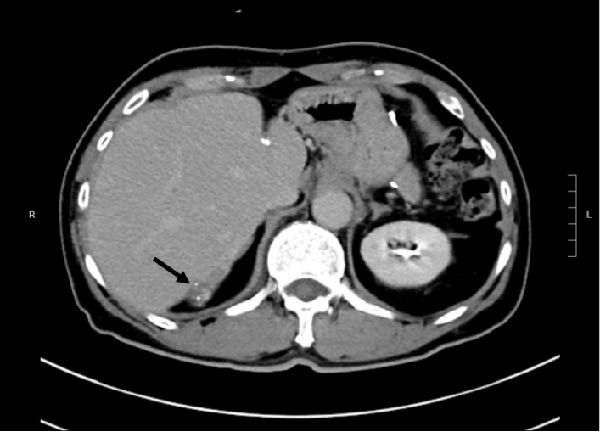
CT showing a small recurrent tumor at seg 7 (arrow).
